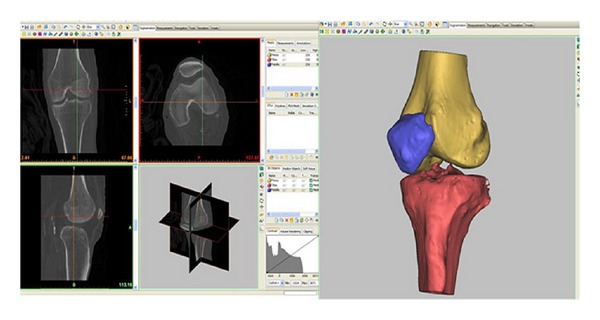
Segmenting process rendering CT scan into 3D model of the knee.
